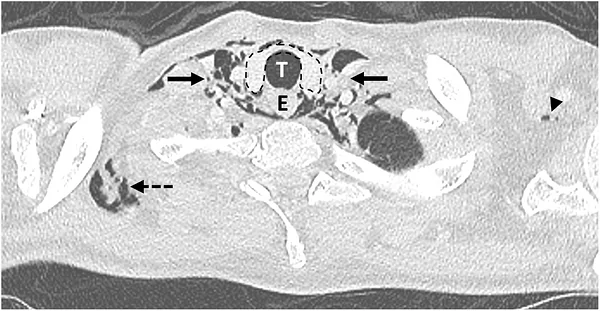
Axial CT (lung window) at the level of the inferior neck shows upward dissection of pneumomediastinum into: the soft tissues of the anterior neck (black arrows), surrounding the thyroid gland (hashed outline), trachea (T), oesophagus (E), the right posterior triangle (hashed arrow) and left axilla (arrowhead).
